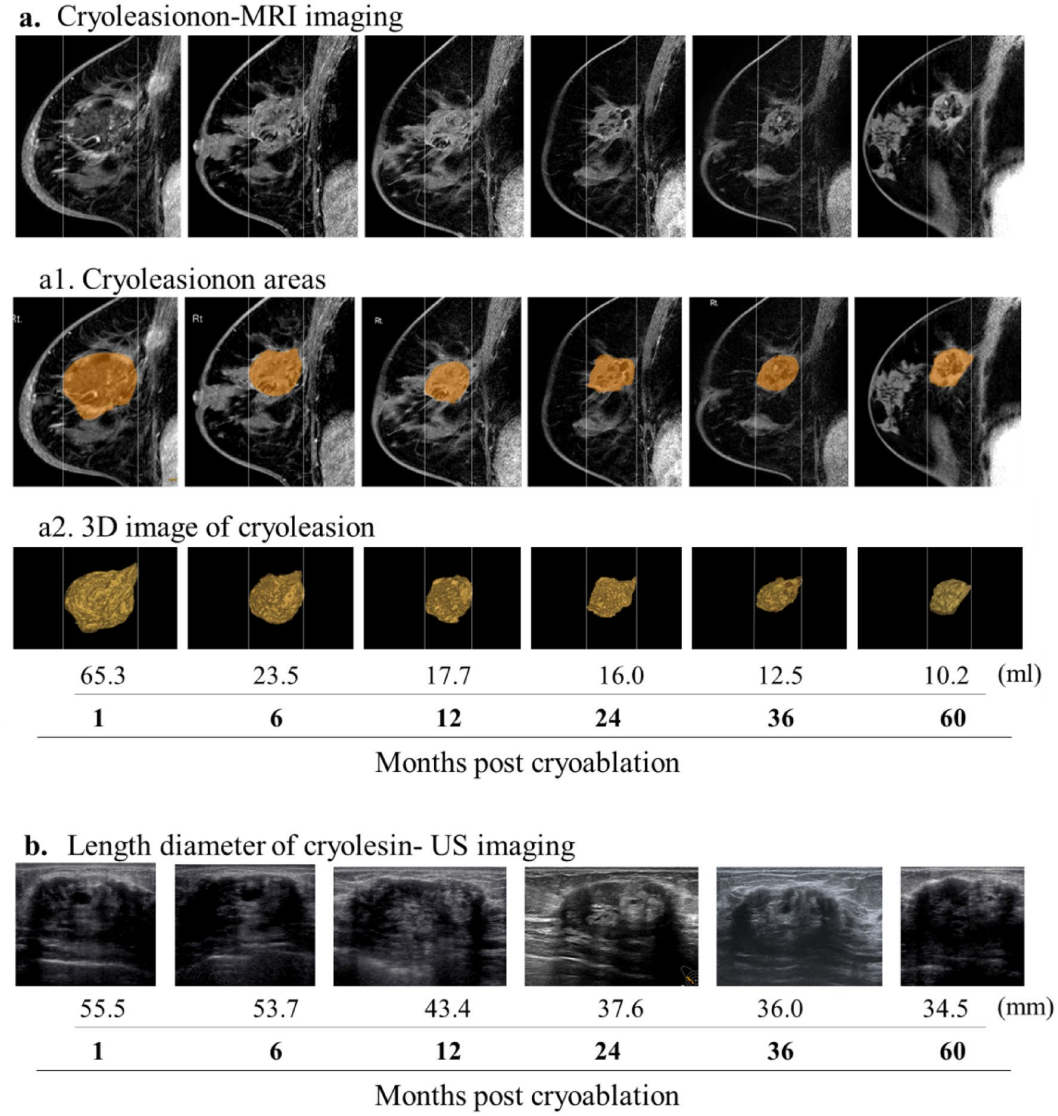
Cryolesion measurements by MRI and US imaging. Post-cryoablation cryolesion images of a patient 67 years old with 12.2 mm tumor in the right breast. Cryolesion’s change over time, as observed by MRI (a) were marked (a1) and measured, and their volume was calculated (a2) using Attractive Basic 3D. Cryolesion as observed by US (b)
